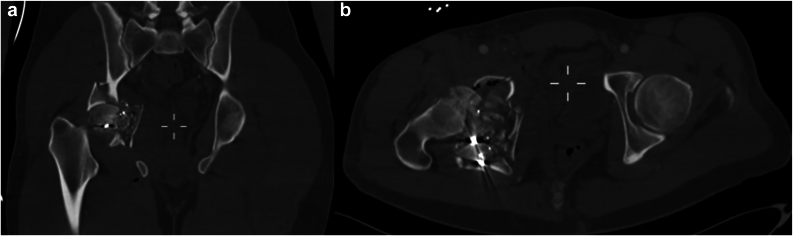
CT scan images in (a) coronal and (b) axial views of the right acetabulum and femoral head upon admission, showing severe comminution of the right proximal femur and acetabulum. Note the metal shrapnel from the high velocity projectile within the comminution (bright white dots). CT, computed tomography.
